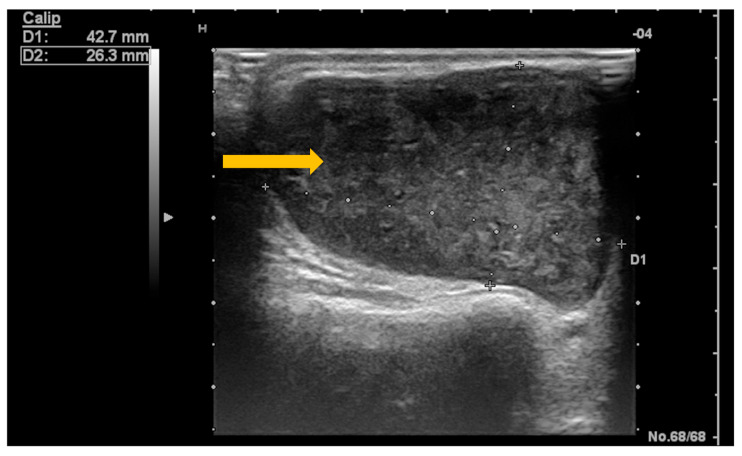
B-mode ultrasound of the parotid gland in a patient with Sjögren’s syndrome. Gray-scale (B-mode) ultrasound demonstrates a newly developed hypoechoic nodular lesion within the parotid gland (arrow), a finding suspicious for salivary gland lymphoma in the clinical context of Sjögren’s syndrome.
